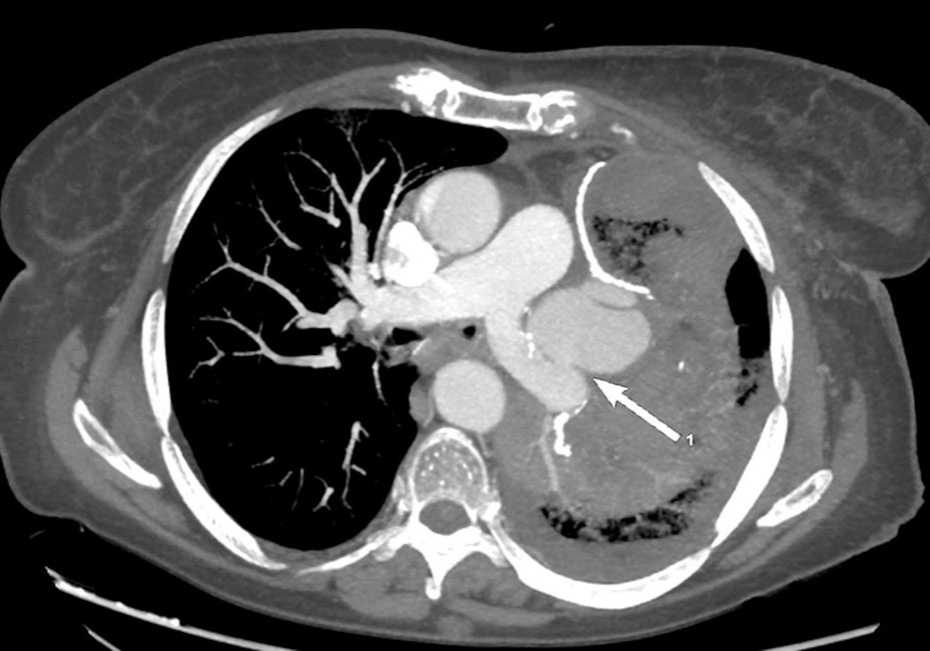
Axial chest computed tomography angiography shows pulmonary artery pseudoaneurysm (white arrow) surrounded by lung parenchymal consolidation.
